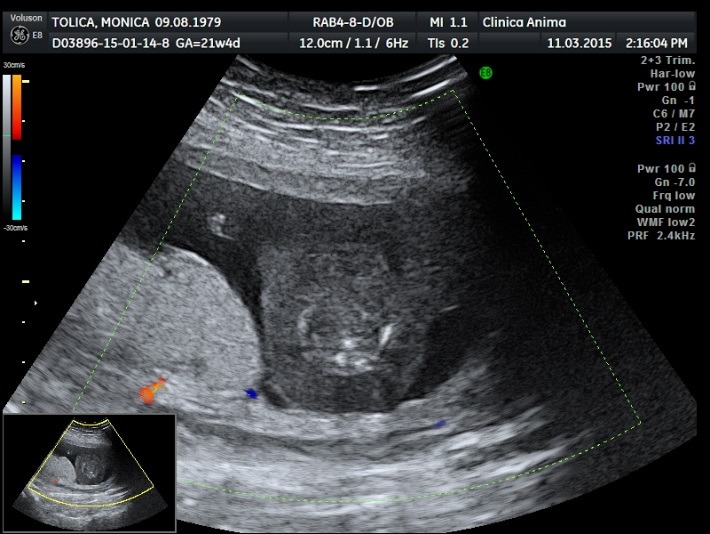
Involution of the tissular mass growth and lack of Doppler signal
